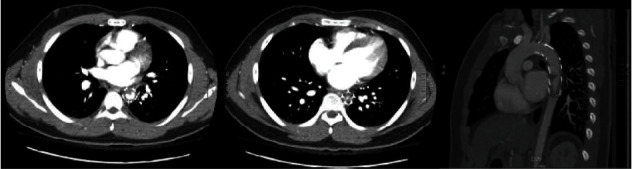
CT scan revealing an endovascular thoracic aortic stent graft spanning the distal arch through the mid descending thoracic aorta. Beginning at the distal third of the stent is circumferential low-attenuation centrally progressing to near complete occlusion at the terminal portion of the stent. Near occlusion at the approximate level of the T7 and T8 intervertebral disc. The superior portion of the thrombus demonstrates a triangular morphology. Small renal infarcts are also noted, seen in the sagittal plane.
